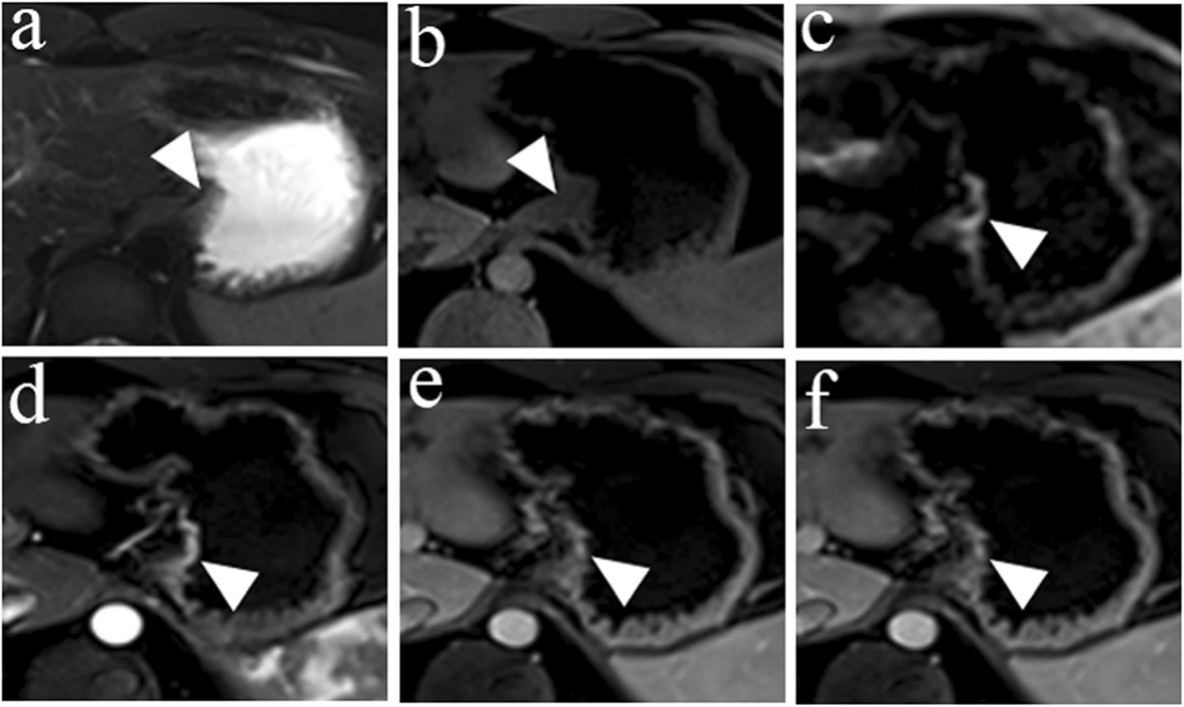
A 24-year-old man with esophagogastric junction cancer pathologically diagnosed as stage T1a, Sierwert III and was performed using conventional MRI. a The axial T2W image shows a lack of obvious thickening of the cardia wall. b The axial diffusion-weighted image with b = 800 s/mm^2^ shows hyperintensity in the cardia wall. c No thickening pattern of the cardia wall was shown in the axial T1W image. d The arterial phase of the enhanced T1W image shows a marked enhancement of the lesion. e, f Compared with the adjacent normal tissues, the lesion showed a similar enhanced pattern on venous and delayed phases of enhanced T1W images
